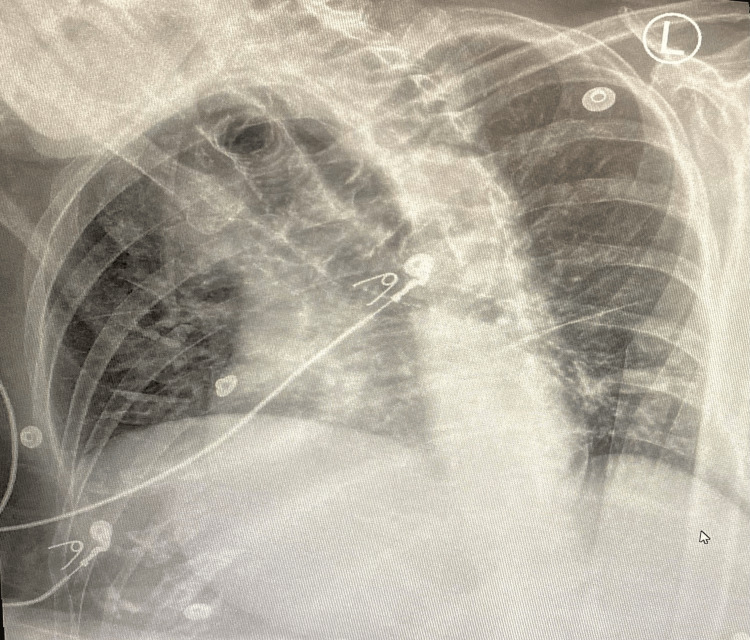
Chest X-ray of the patient The chest X-ray failed to reveal any consolidation
